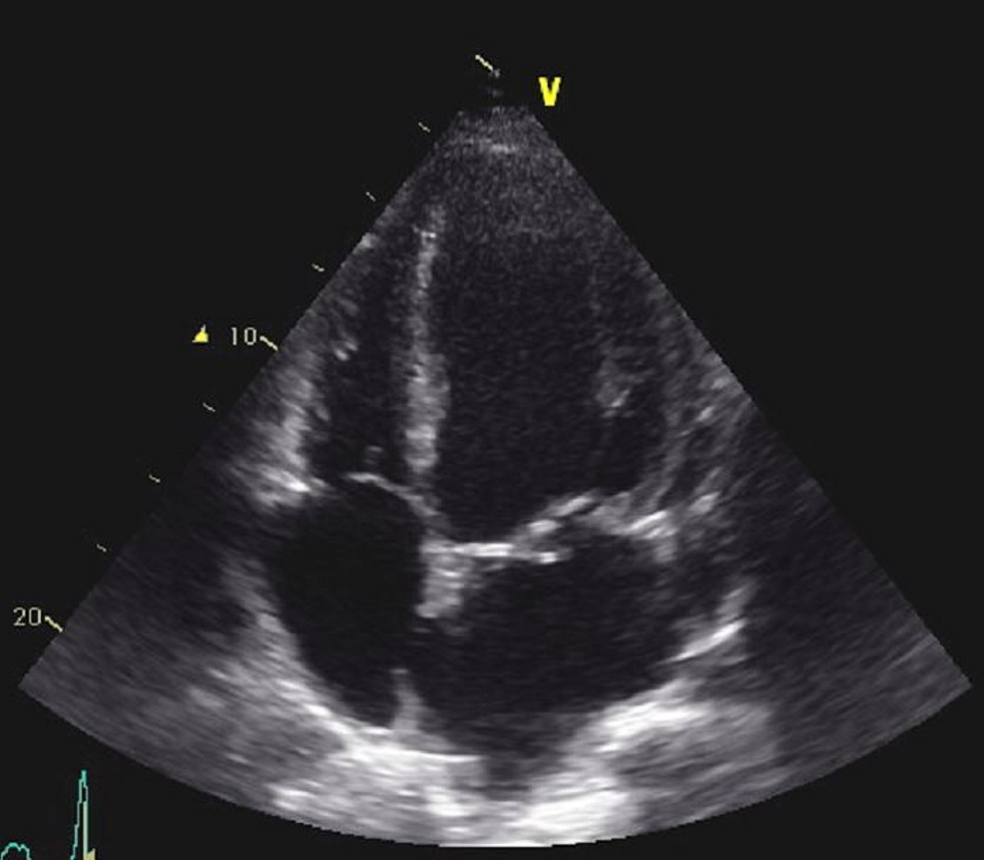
Apical four-chamber view of the heart showing mitral valve prolapse.
